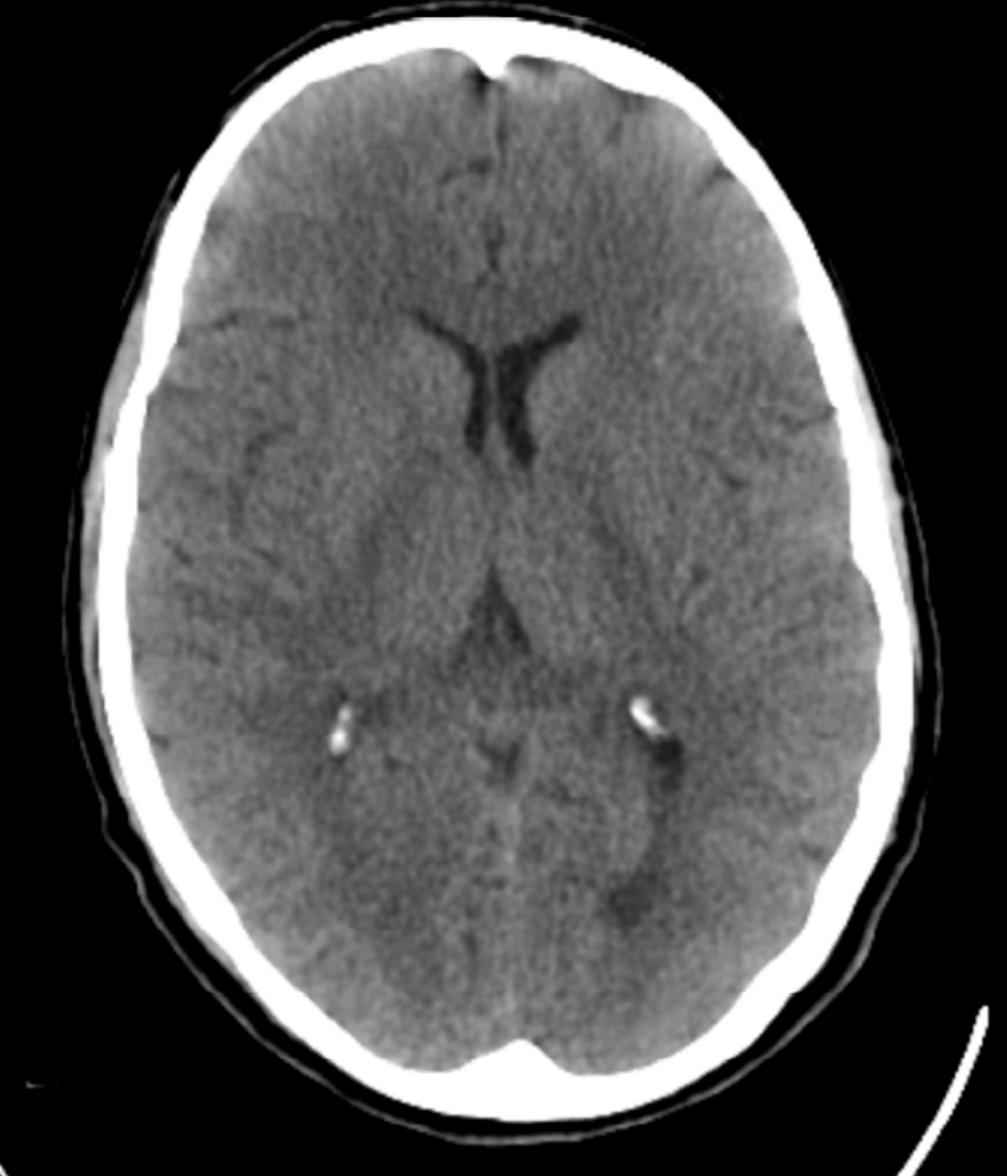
Head CT (non-contrast) Findings: No intracranial bleed or mass. No midline shift. The basal cisterns are patent. No orbital collection or fracture. Minor mucosal thickening in the paranasal sinuses. Mastoid air cells are clear. Conclusion: No intracranial bleed. No intracranial mass.
